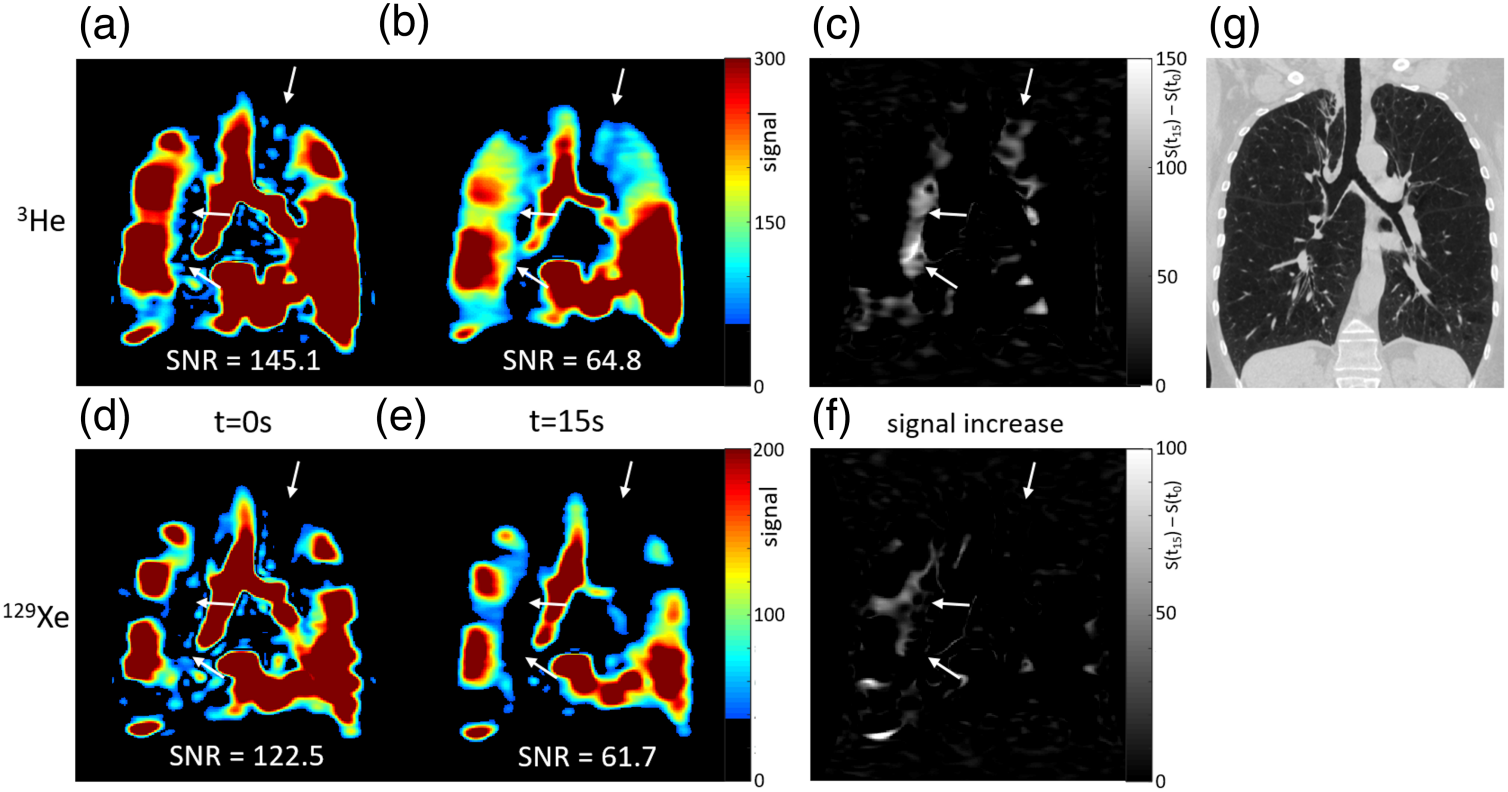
^3^He images (top) and ^129^Xe images (bottom) acquired from patient 2 during breath‐hold; (a,d) at the start of the imaging sequence, t = 0 sec, and (b,e) after 15 seconds, both shown with the same signal range. (c,f) Maps of signal increase from t = 0 sec to t = 15 sec. White arrows highlight regions of lung where ^3^He signal increased over time, but ^129^Xe signal did not. (g) CT showed severe centrilobular emphysema and severe hyperinflation, and all fissures appeared intact. Mean SNR over the whole lung ventilated volume for that timepoint is displayed for a, b, d, and e.
